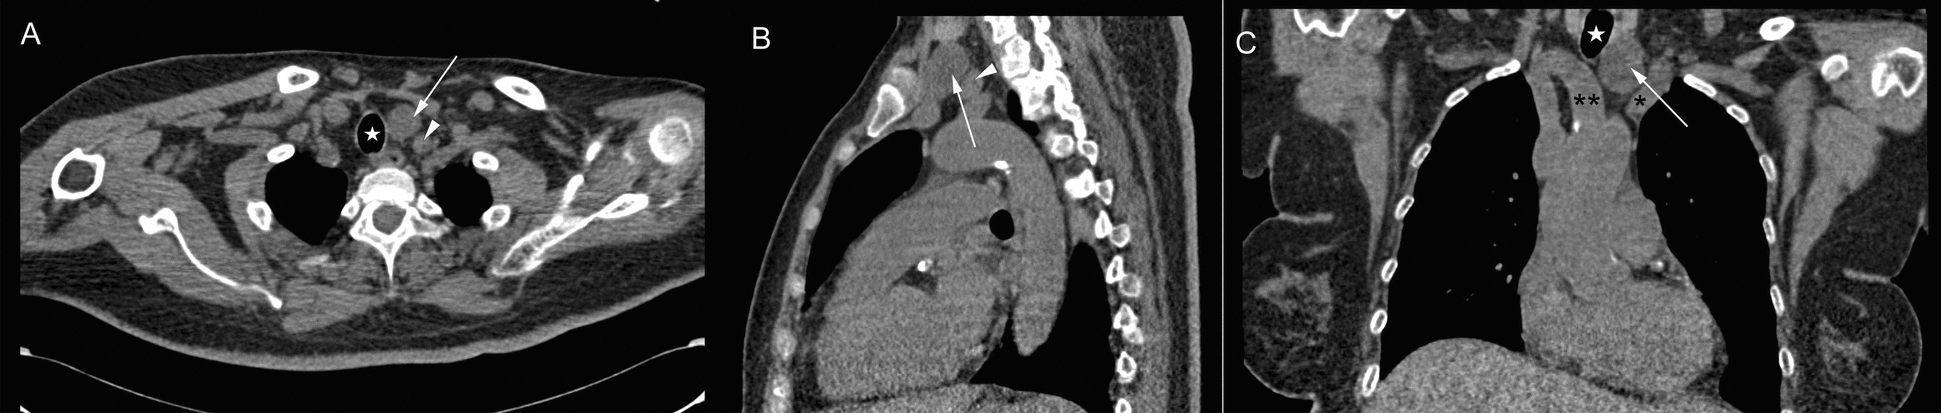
Neck computed tomography scan on admission day. Axial (A), sagittal (B), and coronal (C) computed tomography showing a round hypodense lesion (arrows) between the trachea (stars) and the left carotid artery (arrowheads); asterisk, left brachiocephalic vein; double asterisk, brachiocephalic trunk
